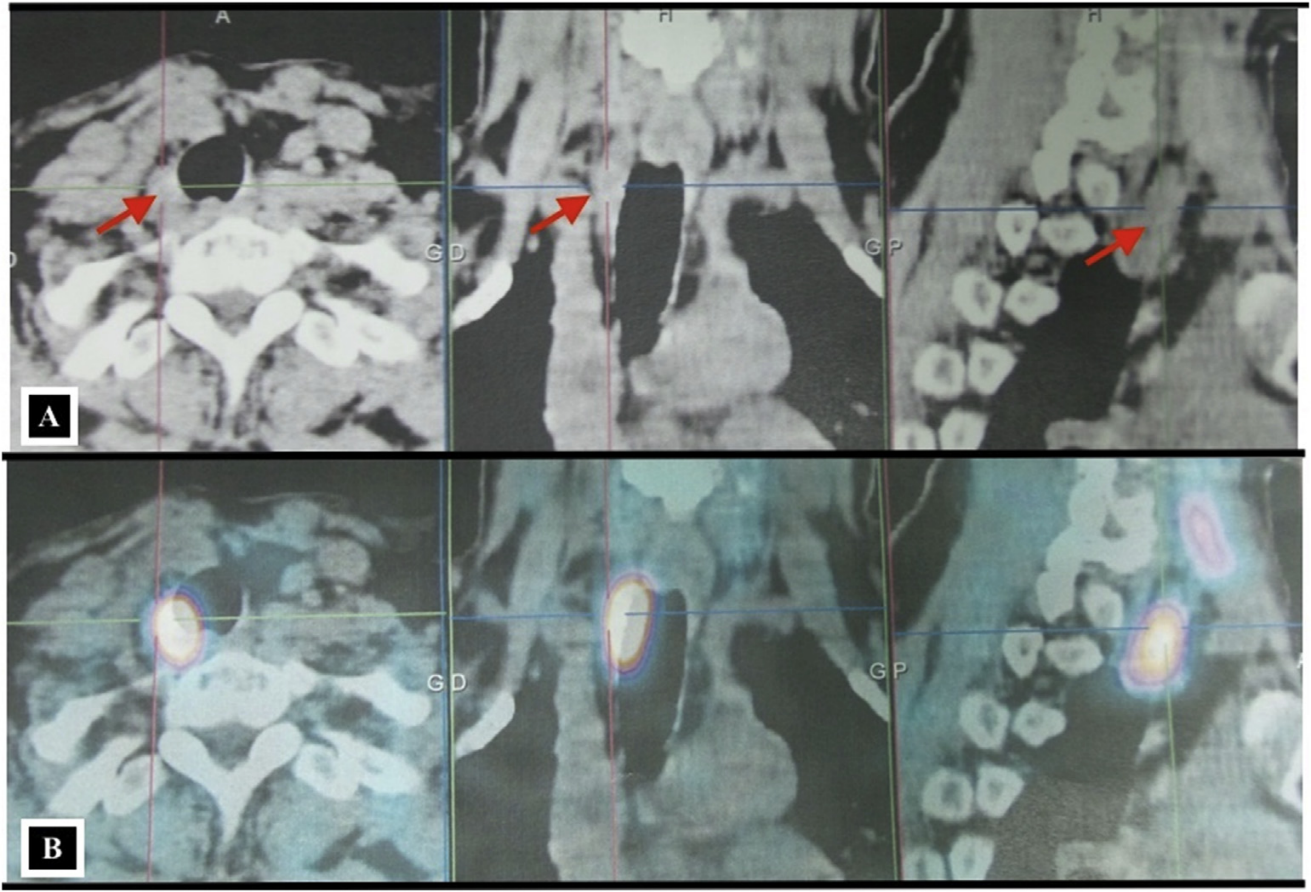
A: Cervico-thoracic CT-scan showing a right latero-tracheal mass that is spontaneously hyperdense. B: On sestamibi-scan (99 m Tc – MIBI) this mass shows an important tracer uptake.
